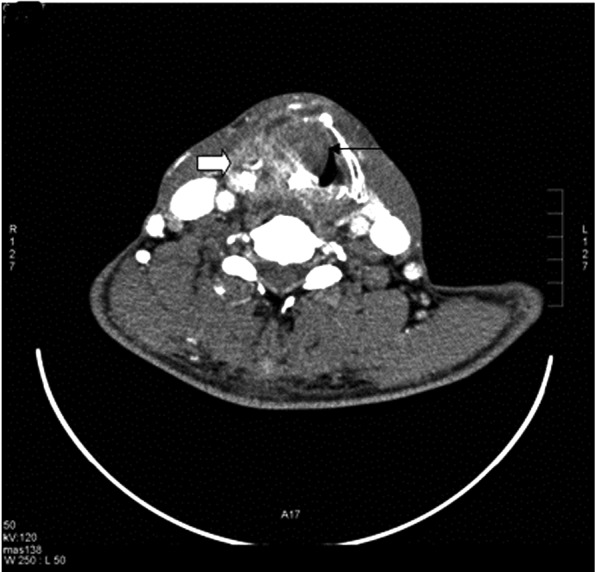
Computed tomography (CT) scan showing a thyroid carcinoma and a laryngeal carcinoma.
